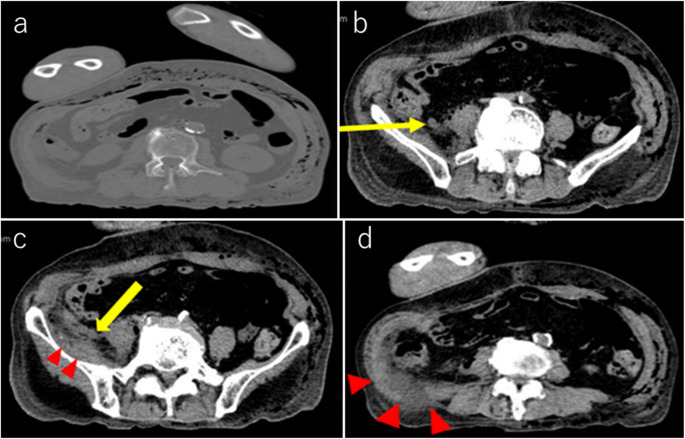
Axial view on postmortem computed tomography. a Free air was present in the subcutaneous abdominal region. b The appendix (yellow arrow) was observed in the right upper abdominal region. c A low-density area (red arrowhead) was found in the retroperitoneum. The appendix (yellow arrow) was observed next to the iliopsoas muscle. d A low-density area (red arrowhead) was found in the retroperitoneum
